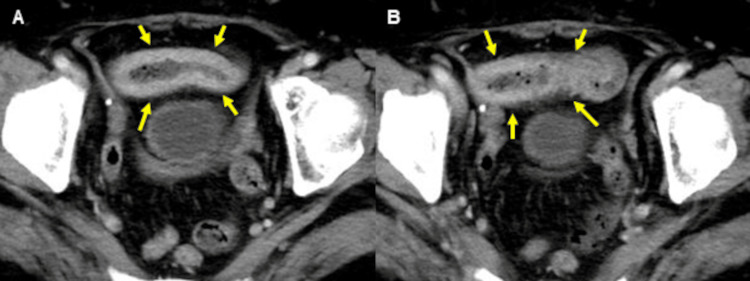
Computed tomography (CT) reveals focal wall thickening in the ileum.
